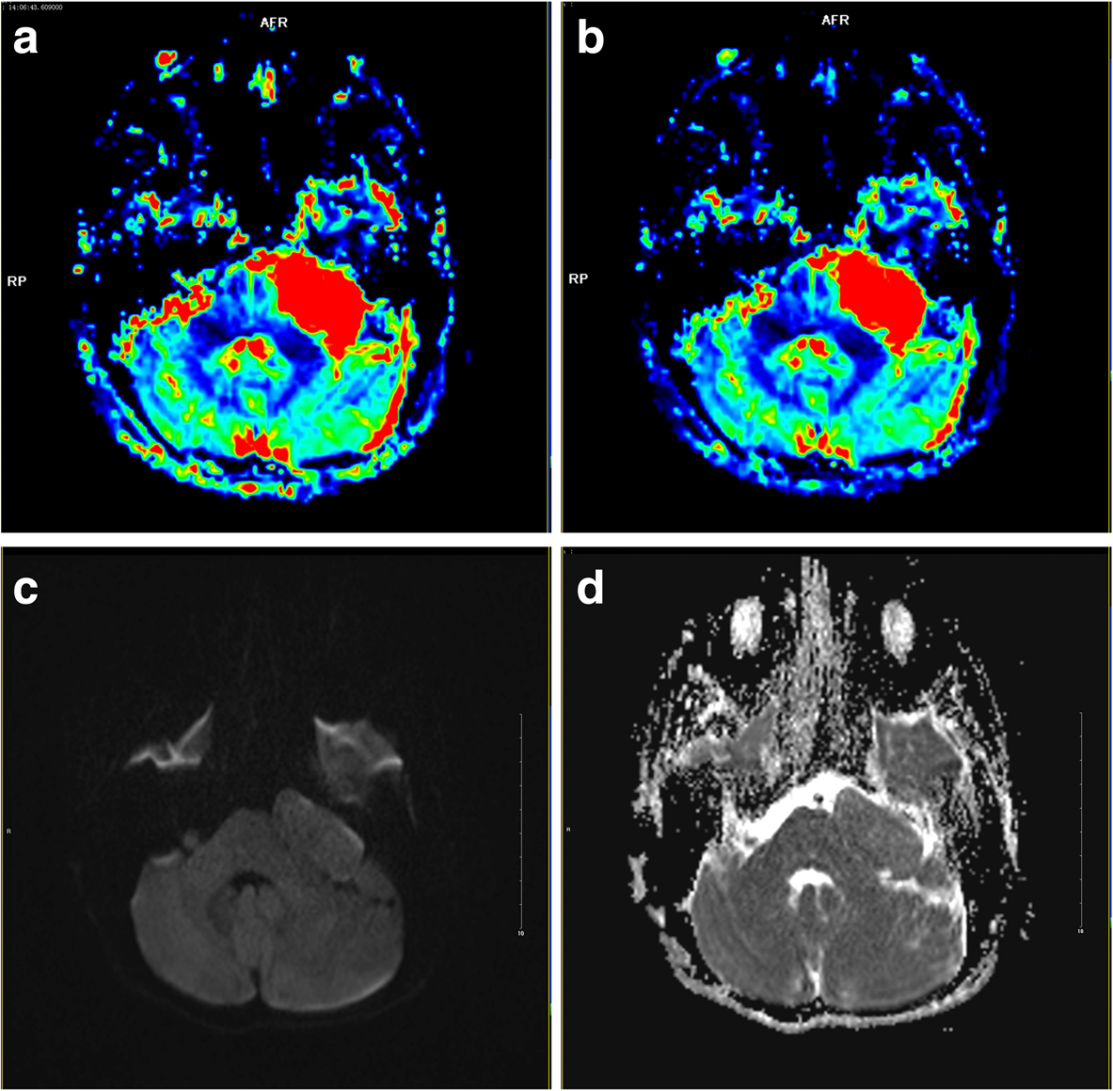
rCBV and rCBF on DSC perfusion, DWI and ADC map of trigeminal ganglioneuroma. The tumor showed increased rCBV (a) and rCBF (b), and homogeneous isointense on DWI (c). and higher ADC value on ADC map (d) compared with adjacent brain tissues
